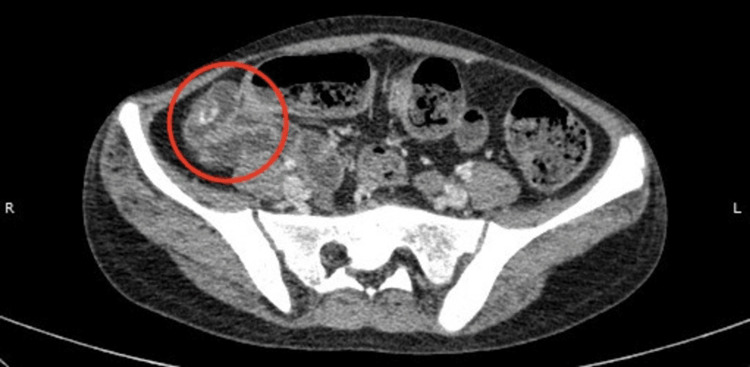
CTA of the abdomen showing bowel hemangiomas A computed tomography angiography (CTA) of the abdomen was planned. The red circle highlights a few intramural polypoidal lesions with intraluminal growth patterns involving the distal ileum and descending colon. These lesions show heterogeneous enhancement in the venous phase; a possibility of bowel hemangiomas was suggested
